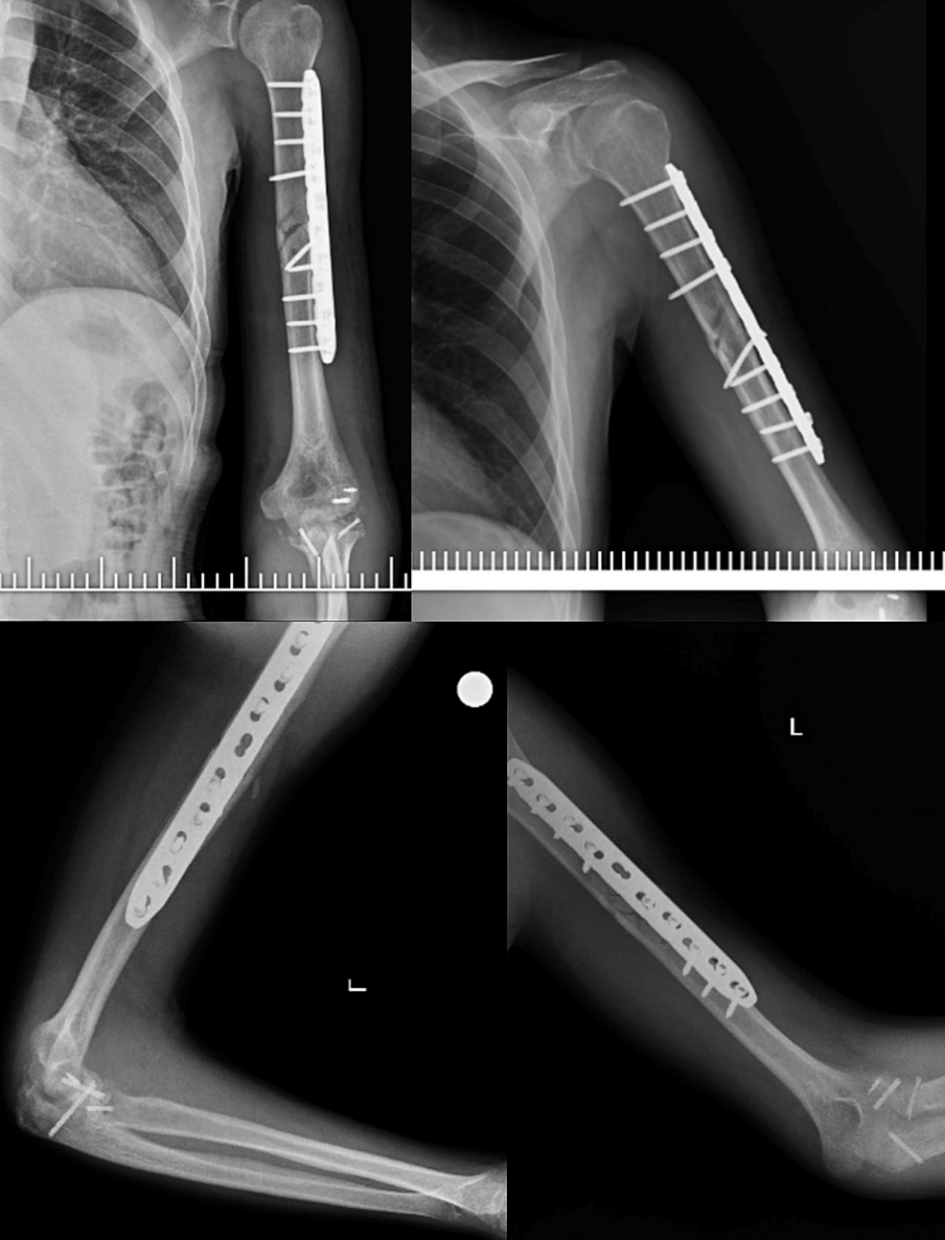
Late follow-up X-ray views demonstrating osteosynthesis of humerus shaft, capitellum, coronoid, and radial head fractures.
